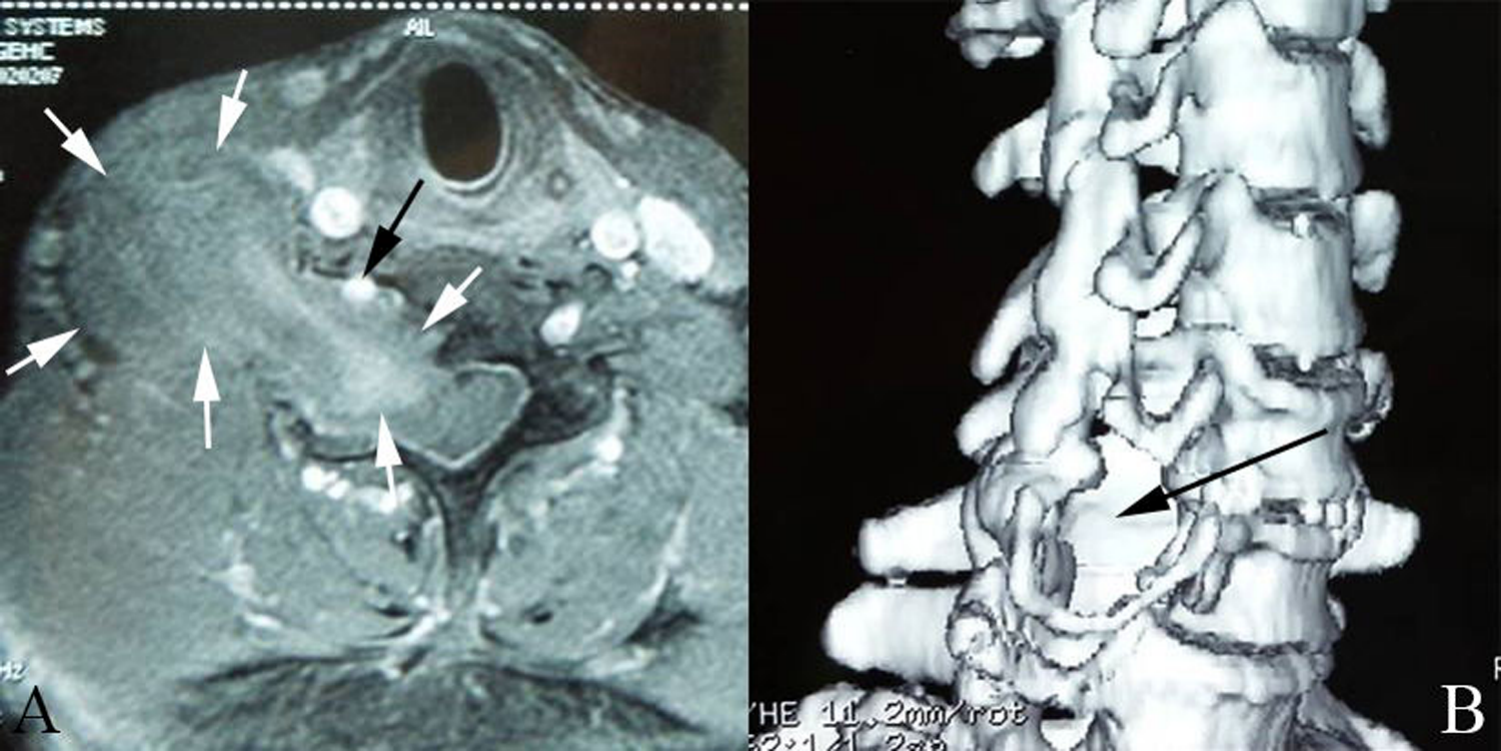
(A): the vertebral artery was displacedanteriorly and partially encircled by the tumor. White arrow: tumor; Black arrow:vertebral artery (B): Three- dimensional CT reconstruction shows intervertebral foramen was obviously enlarged (black arrow).
